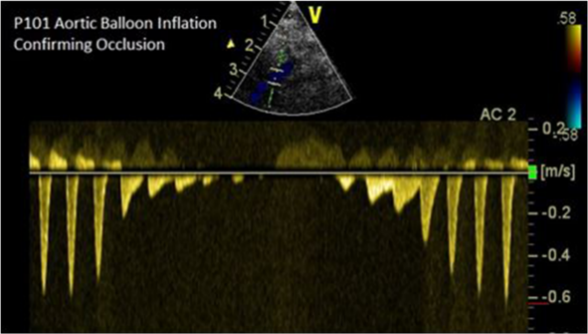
Spectral Doppler (SD) flow confirmation of “tourniquet” function of the intra-aortic balloon. Flow in the femoral artery was eliminated by balloon inflation and returned rapidly with balloon deflation. SD evaluation was repeated following therapy
